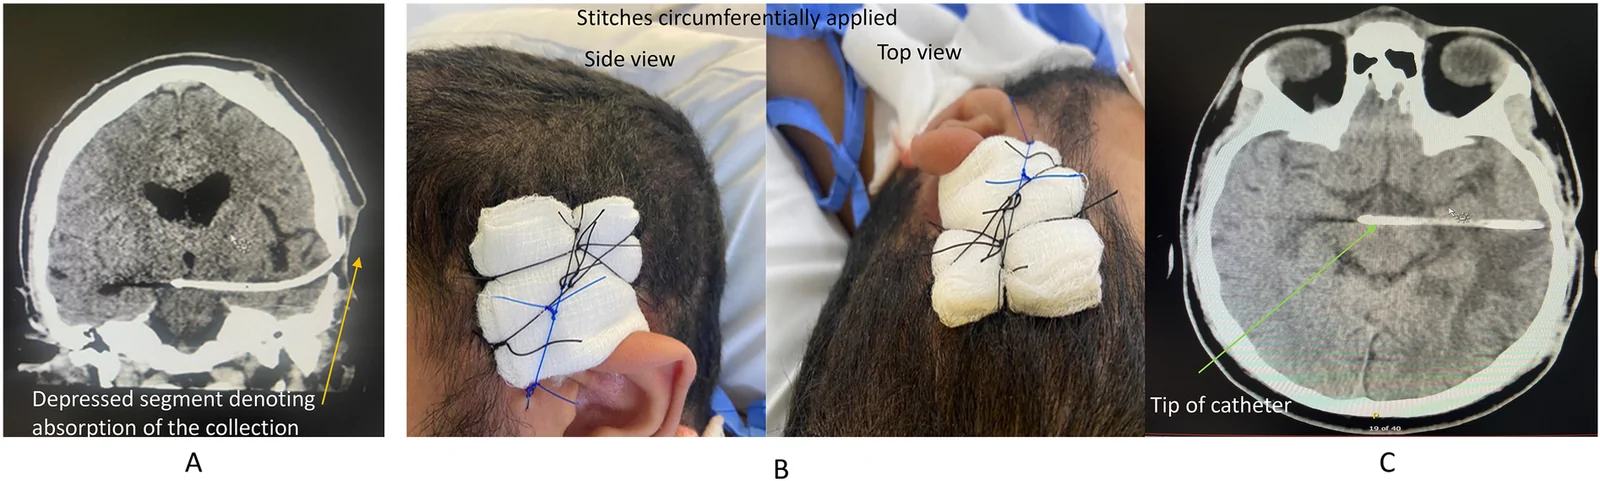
(A) CT Scan coronal view of the brain following gauze application technique showing subside of the collection with significant improvement in comparison to the pre op scan. Arrow (yellow) pointed toward the depression due to fixed applied gauze. (B) Images demonstrating the application of stitched dressing gauze circumferentially over the wound, tight by silk and proline stitches at the corners in both directions, craniocaudal and mediolateral, with a sealing effect. (C) CT Scan axial view of the brain four years follow-up showing subside of the collection with no recurrence and tip of catheter remain in place (green arrow).
